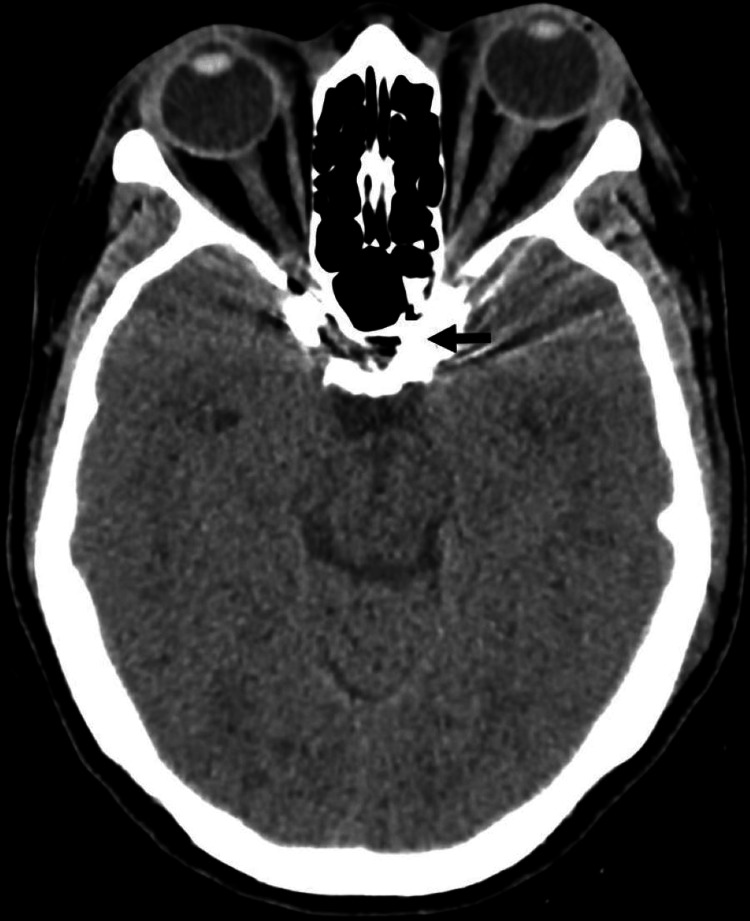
Axial non-contrast CT brain showing coils in the region of the left cavernous sinus (arrow) and no post-procedure complications.
